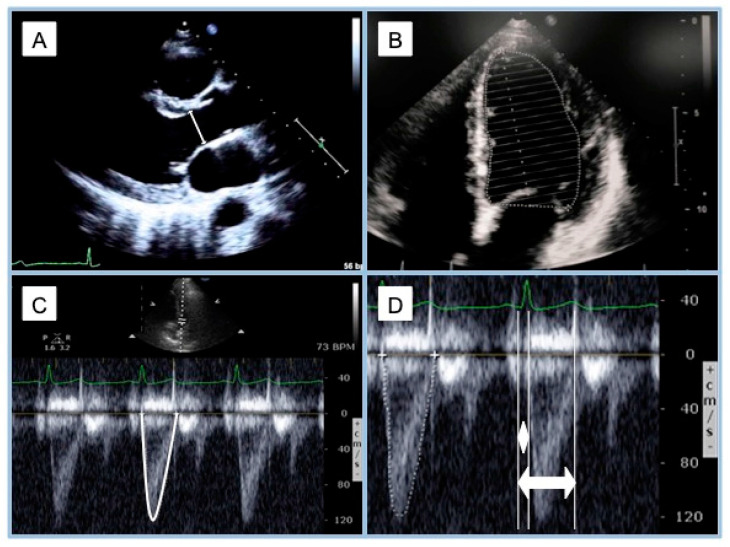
Echocardiographic estimation of left end-systolic elastance using Chen’s method. (A): Parasternal long-axis view, left ventricle outflow tract diameter measure; (B): left ventricle ejection fraction measured by Simpson’s biplane method, apical 4-chamber view in this picture; (C): apical 5-chamber view, the velocity–time integral of left ventricle outflow tract pulsed-wave Doppler; (D): apical 5-chamber view, left ventricle outflow tract pulsed-wave Doppler, pre-ejection time (white diamond) and total ejection time (white double arrow).
